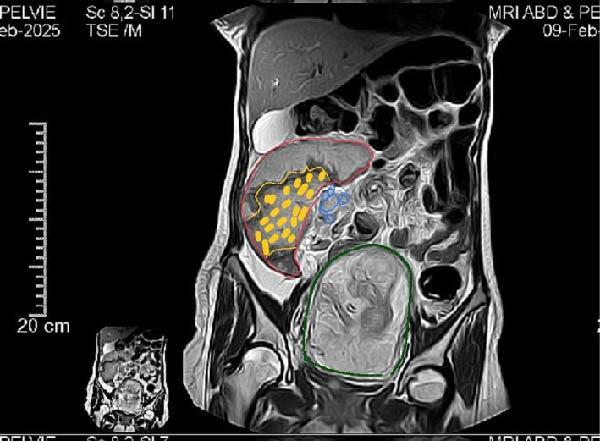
MRI findings of wandering spleen with infarction and splenic vein thrombosis, (green) represents gravid uterus, (blue) represents aneurysmal dilatation of splenic vein stagnant flow, (red) represents enlarged wandering spleen, (yellow) represents splenic infarct.
